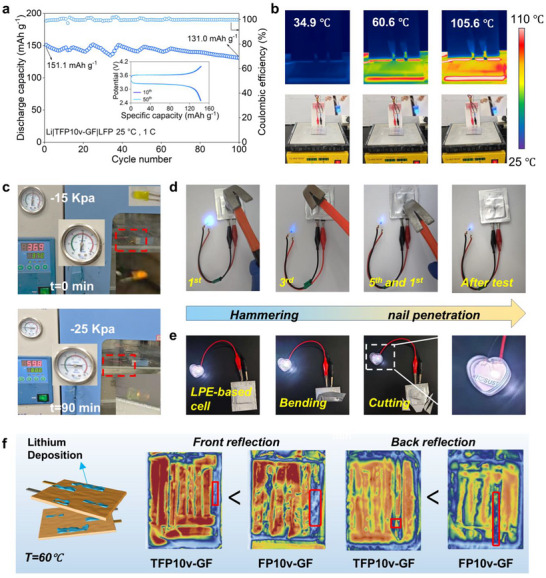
Safety evaluation of Li|TFP10v‐GF|LFP pouch cell. (a) Cycling performance of TFP10v‐GF‐based (Li||LFP) pouch cell at 25°C, and the inset is the corresponding charge‐discharge curves. (b) Thermal stability test. (c) vacuum‐heating test in a vacuum environment of 60°C. (d, e) Digital photographs of pouch cells operating under harsh mechanical abuse, multiple folding, needle puncture tests, and cutting. (f) Static ultrasonic testing of Li|TFP10v‐GF|LFP and Li|FP10v‐GF|LFP pouch cells.
